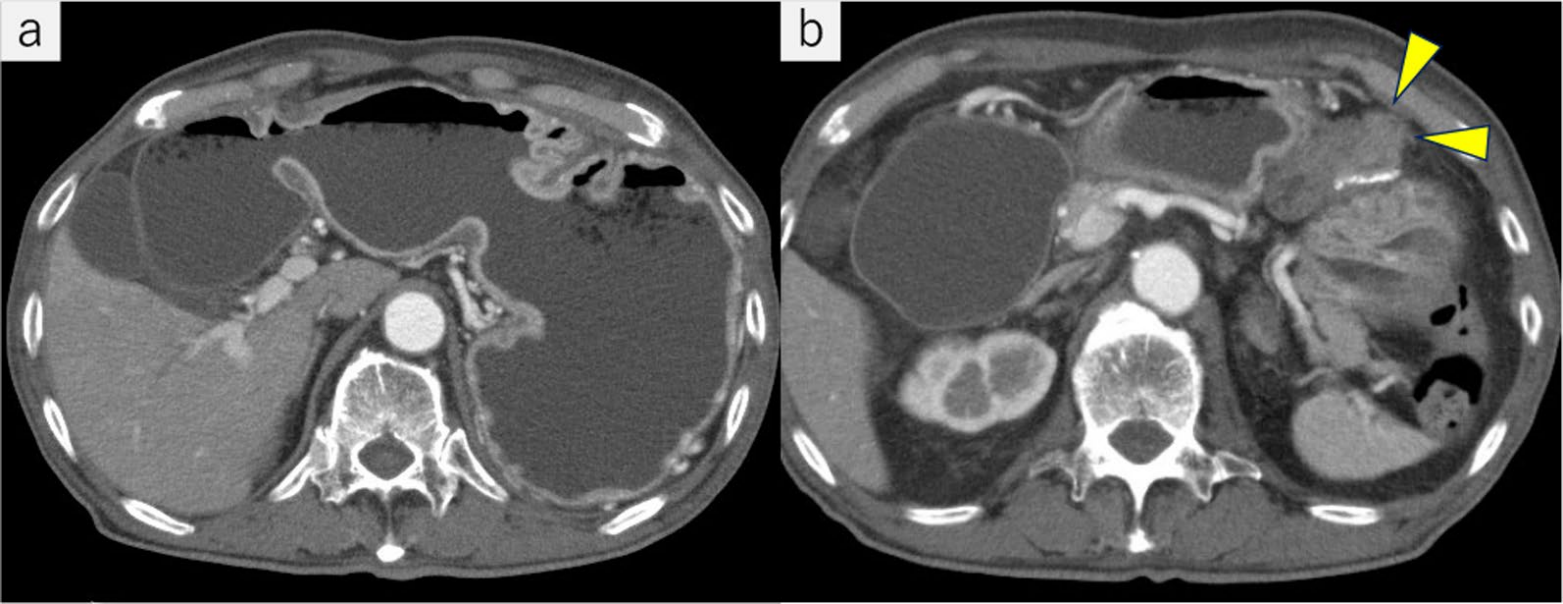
Computed tomography on admission. a Stomach and duodenum are prominently dilated. b Obstruction at the gastrojejunostomy anastomosis was caused by disseminated left renal pelvis cancer (arrowhead)
